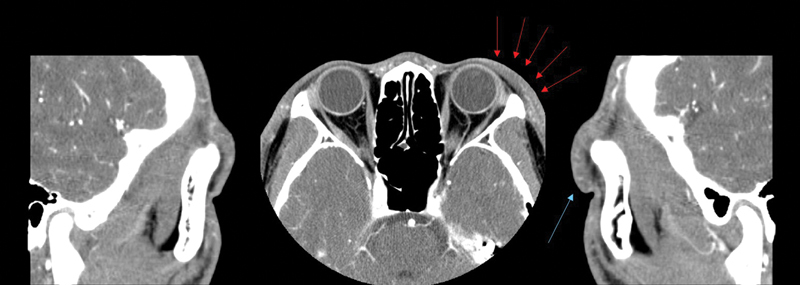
Facial computed tomography scan of a patient with left upper eyelid lymphedema. (Left) Sagittal view of unaffected side without any lesion. (Center) Axial view and (right) sagittal view of the affected side showing diffuse swelling compared to the contralateral side (red and blue arrows).
